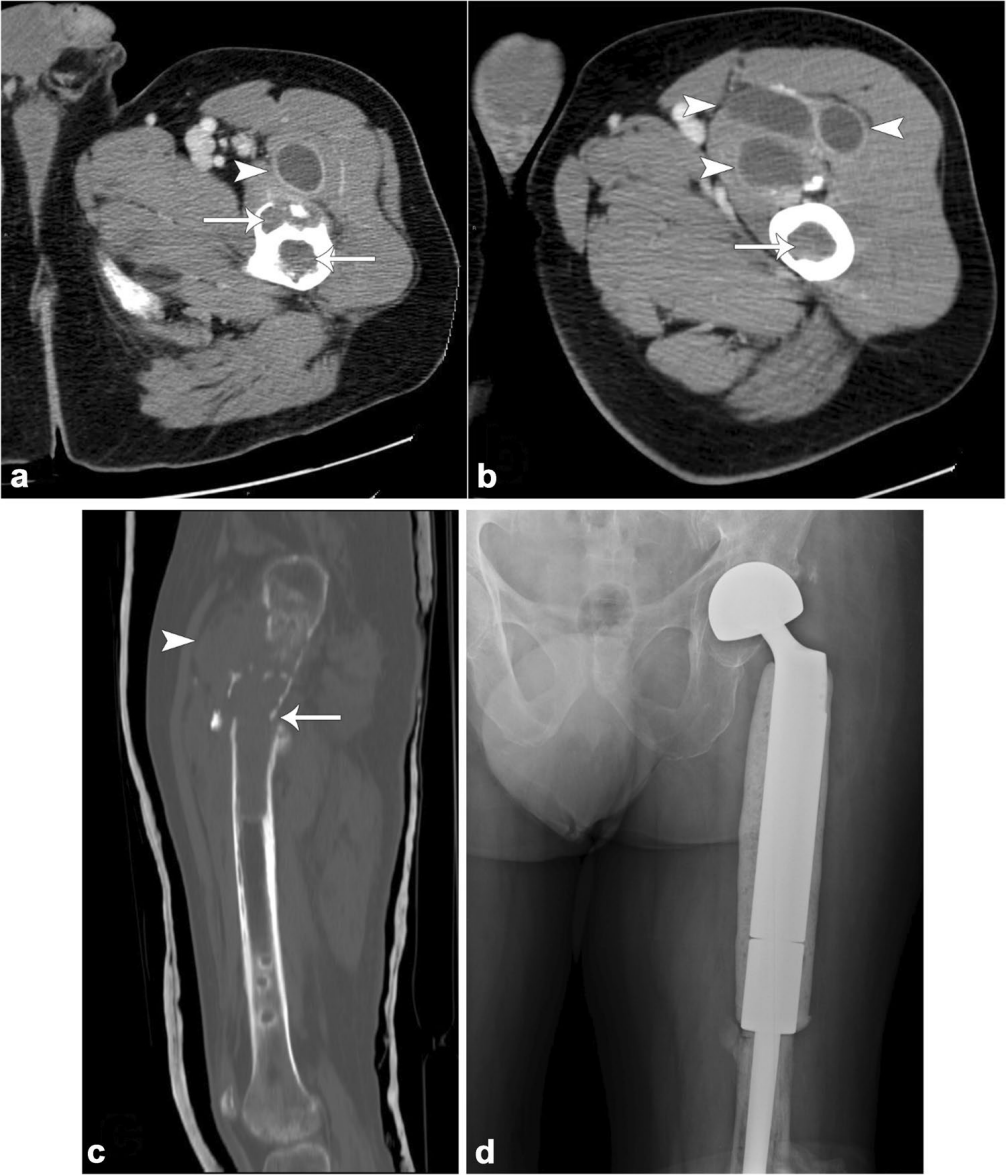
Sixty-year-old male farmer and shepherd from Southern Italy with left femur echinococcosis with pathological fracture. Axial (a, b) and sagittal (c) CT images demonstrate a 15-cm lytic lesion of the proximal femur with pathological fracture (*arrows*) and multilocular daughter cysts (*arrowheads*) extending into the vastus intermedius muscle. AP radiograph (d) 20 years later shows that the patient has done well after proximal femur resection and left total hip modular endoprosthesis placement
